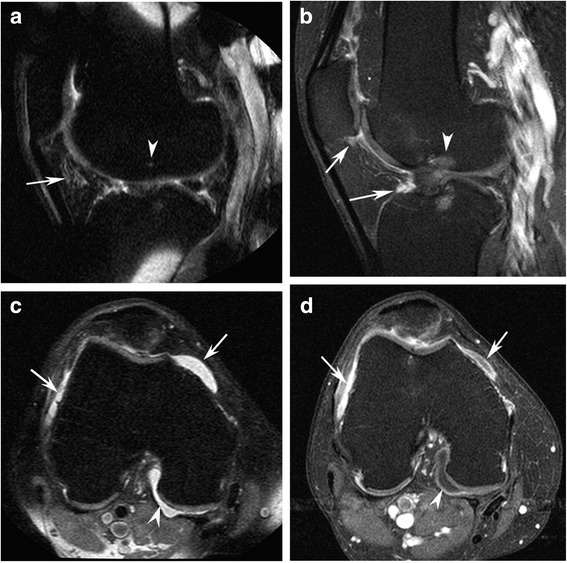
a Sagittal fat-suppressed proton density-weighted image (non-CE-MRI) shows hyperintensity in the intercondylar (*arrow*) region of Hoffa’s fat pad. This signal alteration is used as a surrogate marker for synovitis on non-CE-MRI. There is a discrete subchondral bone marrow alteration in the femur (*arrowhead*) corresponding to the site of the anterior cruciate ligament (ACL) insertion. b In contrast, sagittal fat-suppressed T1-weighted contrast-enhanced MRI (CE-MRI) shows no intercondylar synovitis, but reveals infrapatellar synovitis and synovitis adjacent to the tibial ACL insertion (*arrows*) not seen on non-CE-MRI, as well as bone marrow edema (*arrowhead*). c Axial non-CE-MRI shows a fluid-equivalent signal within the joint cavity suggestive of joint effusion in the peripatellar recesses (*arrows*) and posteriorly (*arrowhead*). d Axial CE-MRI shows marked synovitis anteriorly (*arrows*) and true effusion only depicted posteriorly as hypointensity adjacent to the synovial lining (*arrowhead*). Reprinted from [62] with permission from Elsevier
